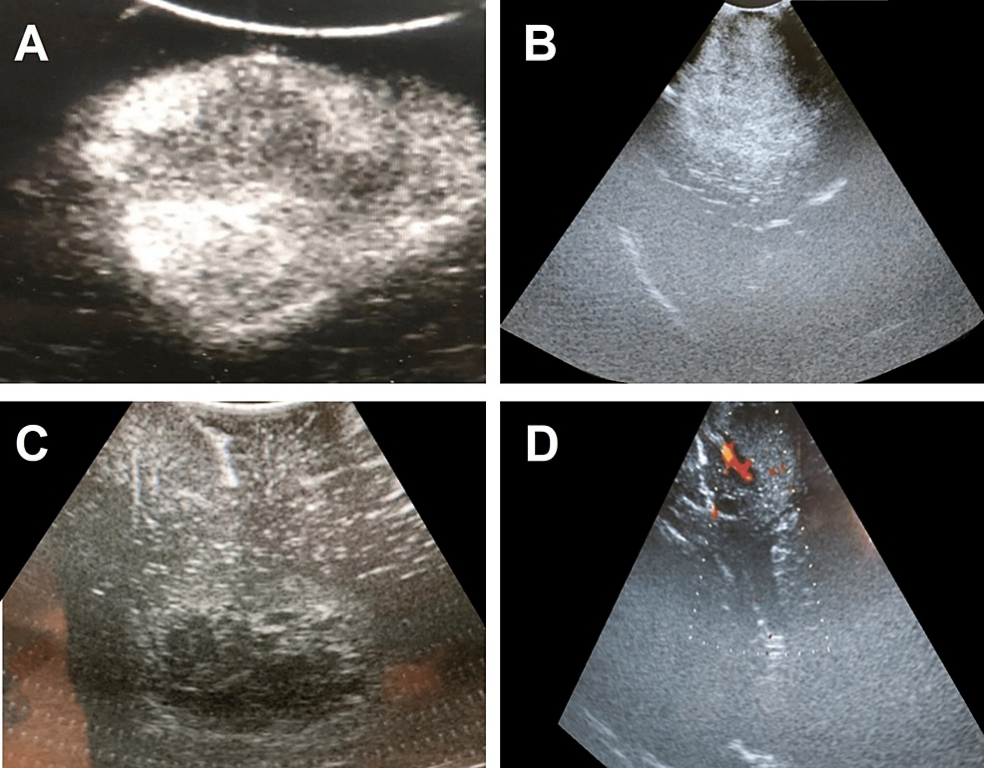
Intraoperative ultrasound images of tumor lesions from patients included in our study. (A) Brain metastasis from breast carcinoma; (B and C) high-grade gliomas; (D) vascularization in a tumor detected using ultrasound
